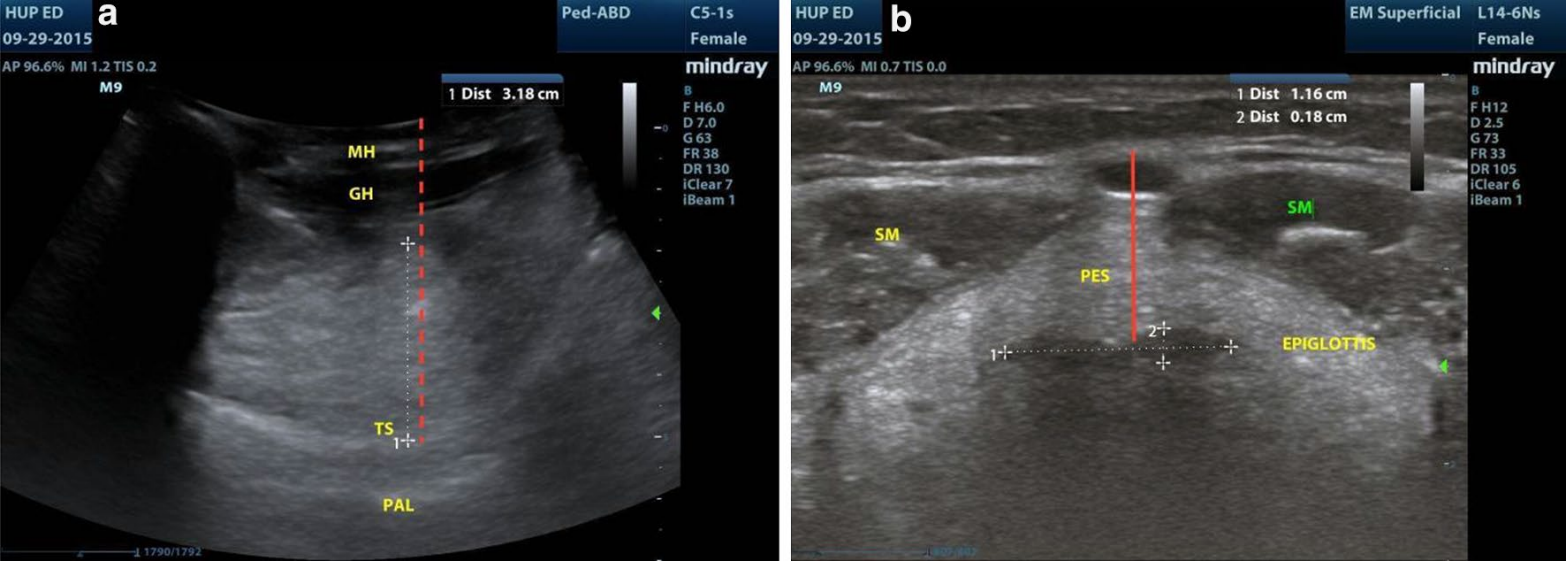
a Sagittal paramedian view of the tongue from the submental area with the white dotted line representing the tongue base measurement and the red dotted line as tongue base-to-skin thickness. The patient’s face would be in the direction of the right of the screen. *MH* mylohyoid muscle, *GH* geniohyoid muscle, *TS* tongue surface, *PAL* palette. b Transverse ultrasound image at the level of the thyrohyoid membrane. The pre-epiglottic space (red line, PES) is measured from the anterior surface of the epiglottis to the anterior margin of the strap muscles (SM) midway between the hyoid bone and the thyroid cartilage. The epiglottis is indicated by the two white caliper lines showing width (dotted line #1) and thickness (dotted line #2)
